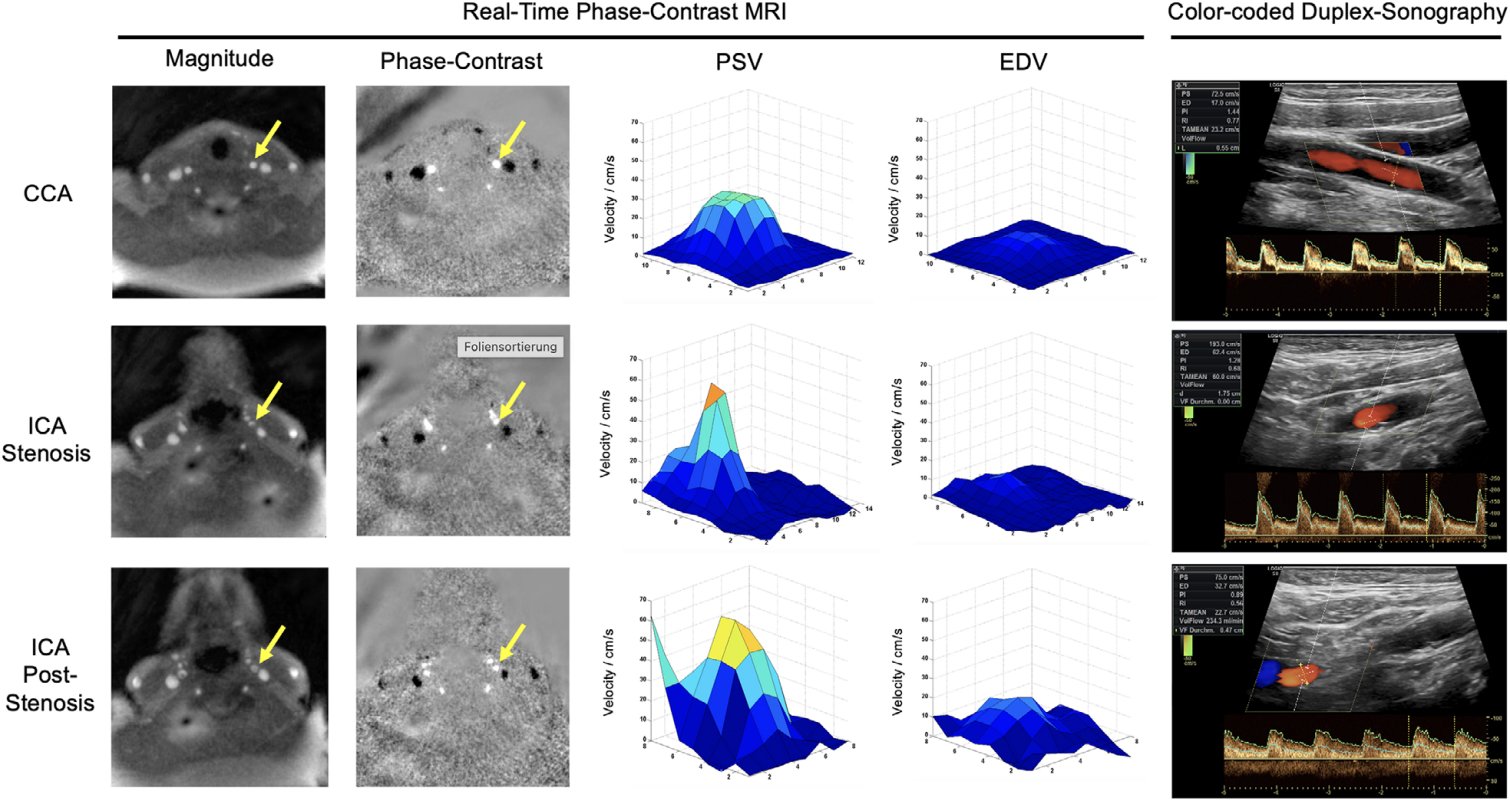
Patient with low‐grade carotid artery stenosis: Representative transversal real‐time phase‐contrast MRI images of the anatomy (magnitude images) and velocity‐coded phase‐contrast images, three‐dimensional representations of the velocities in plane at peak systole (PSV) and end diastole (EDV), as well as representative longitudinal color‐coded duplex sonography images at the common carotid artery (CCA) and internal carotid artery (ICA) at the maximum of the stenosis and post‐stenosis. In the magnitude images, all arteries and veins are hyperintense; in the phase‐contrast images, arteries are hyperintense (upward flow) and veins are hypointense (downward flow). The yellow arrows mark the left CCA, intra‐stenotic ICA, and post‐stenotic ICA. Note the lower pulsatility in the CCA and the higher PSV in the post‐stenotic ICA compared to the patient shown in Figure 2 with a higher grade ICA stenosis. PS, peak systole; ED, end diastole; PI, pulsatility index; RI, resistance index; TAMEAN, time‐averaged mean velocity; Durchm, diameter.
